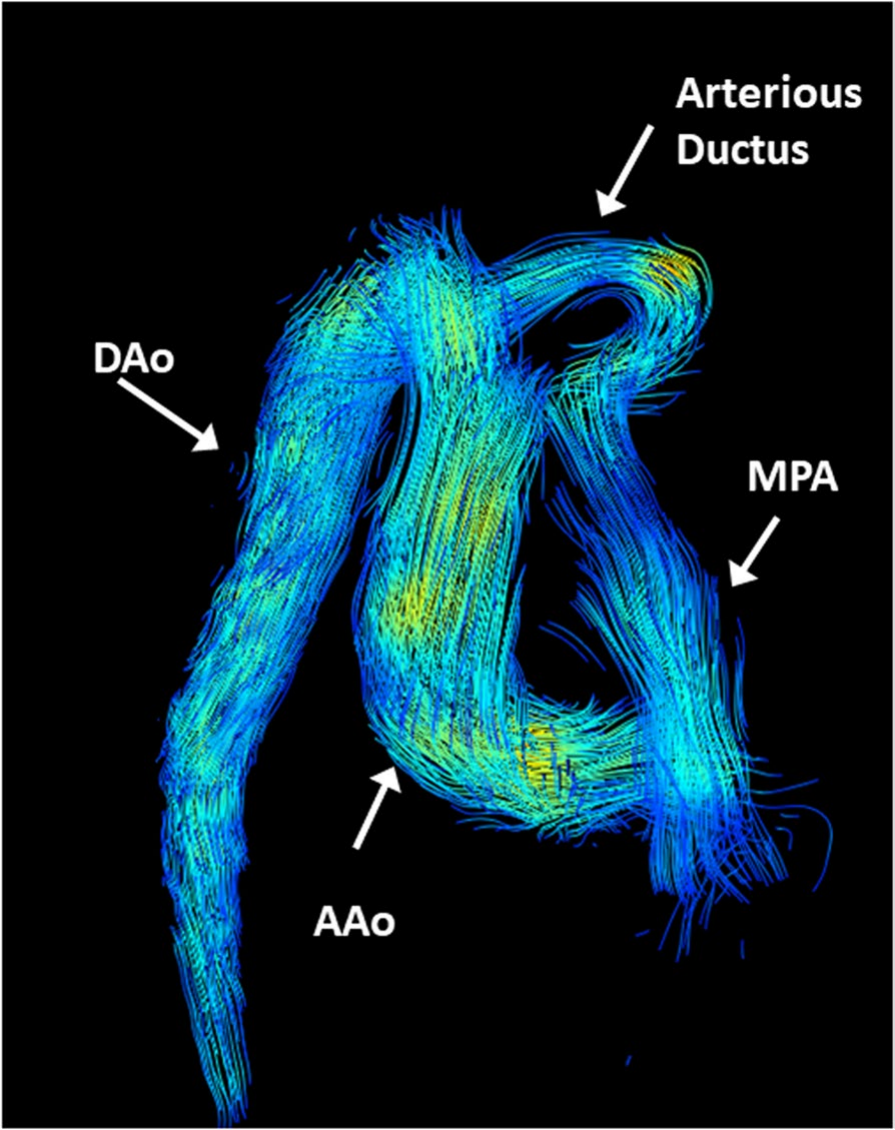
(4D flow MRI): characterization of flow dynamics of the great thoracic vessels (oblique antero-lateral view) in a healthy preterm fetus. Blood flow direction and velocity are indicated by velocity-coded streamlines (DAo descending aorta, AAo ascending aorta, MPA main pulmonary artery, ductus arteriosus). The advent of 4D techniques simplified the demonstration and understanding of even complex vascular anomalies
